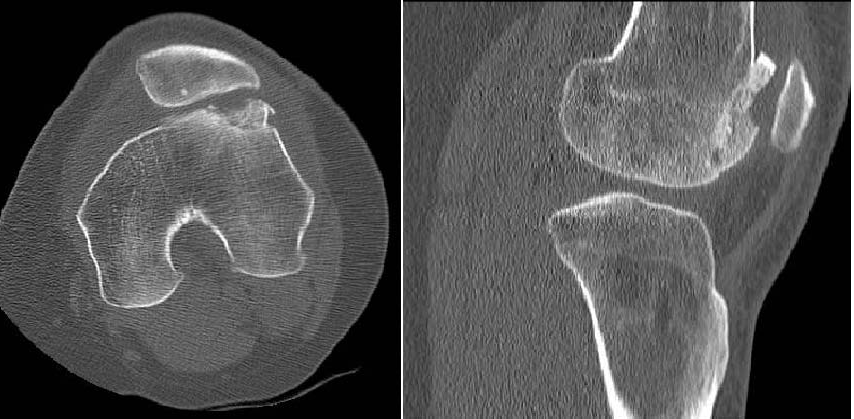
Axial and lateral CT of left knee one year after trochleaplasty demonstrating irregular lateral condyle due to nonunion and collapse of subchondral bone.
